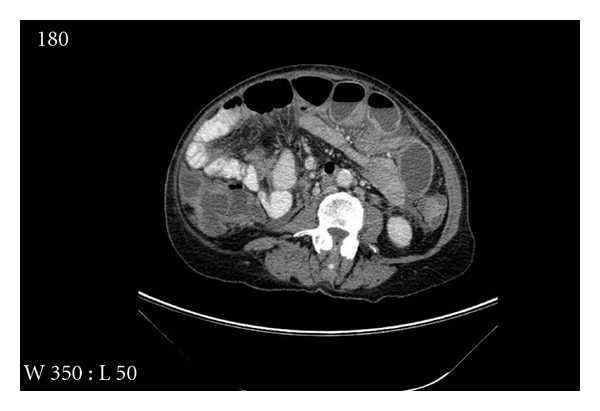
CT scan shows small bowel dilatation, no other specific features.
